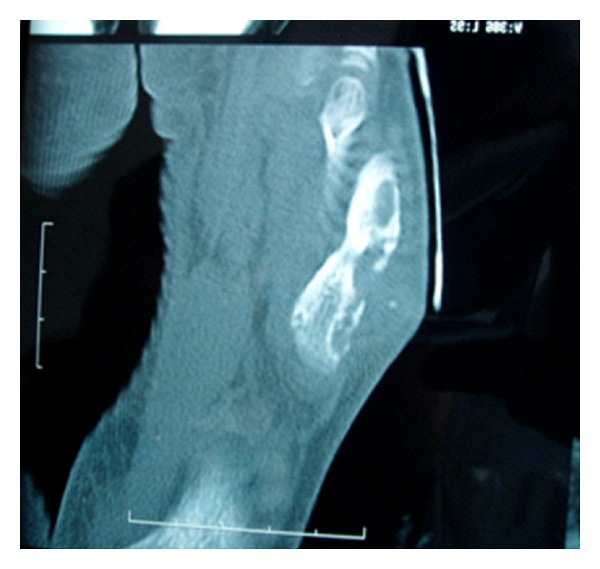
CT showed geodes with reactive sclerosis and soft tissue swelling without collection.
